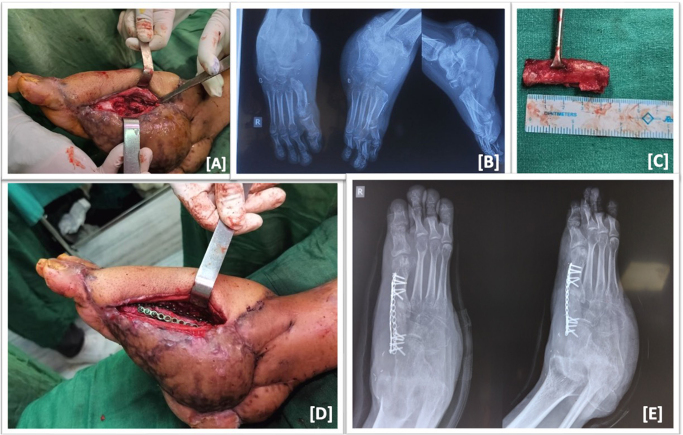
Intra-op clinical picture showing bony defect at 1st tarso-metatarsal joint (A) plain radiograph showing medial arch defect with loss of medial cuneiform (B); 4.0 cm Tri-cortical iliac crest graft was harvested (C); A slot grafting was carried out and fixed with plates and screws (D); post-operative radiographic showing adequate fixation (E).
